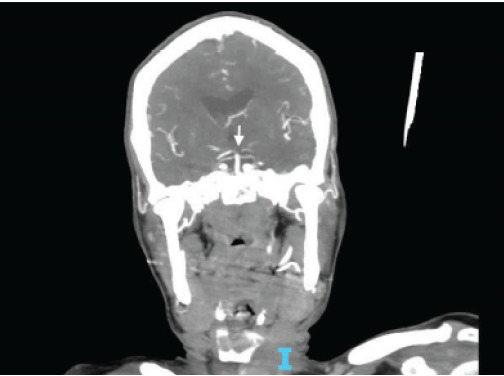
Computed tomography angiography coronal view (Patient 1) with arrow pointing to proximal basilary artery occlusion with multifocal occlusions of the left posterior cerebral artery.
